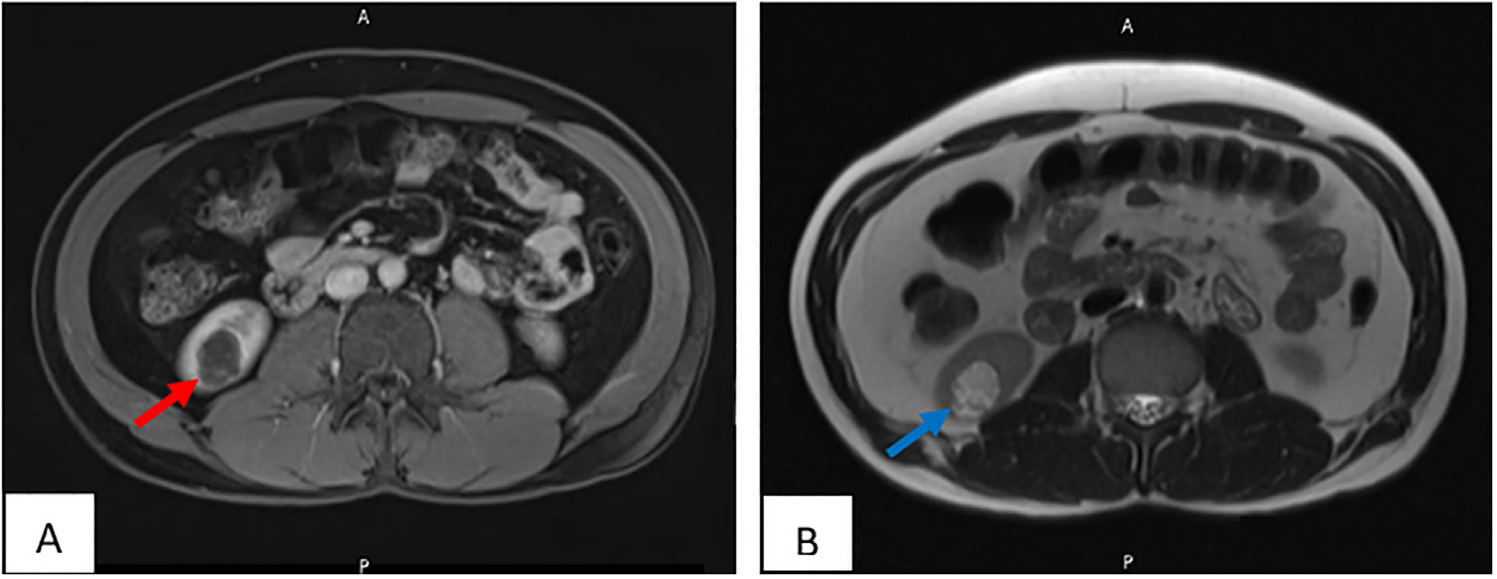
MRI of the right renal lesion, (A) low signal lesion on T1 sequence (red arrow), (B) hyperintense lesion on T2 (blue arrow).
